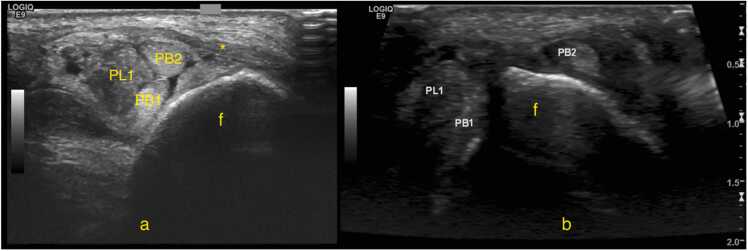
A patient with a history of an ankle sprain 7 months ago. Ultrasound, transverse sections at the level of the lateral malleolus. Section a is slightly higher than section b. Static examination (a), body weight-bearing examination (b). The study revealed a peroneus brevis split tear (PB1 and PB2 – two tendons’ fragments). During weight-bearing, one fragment (PB2) displaced to the lateral contour of the lateral malleolus (f), indicating instability due to damage to the superior peroneal retinaculum (asterisk). The peroneus longus (PL1) is in a normal position but shows signs of tendinosis.
